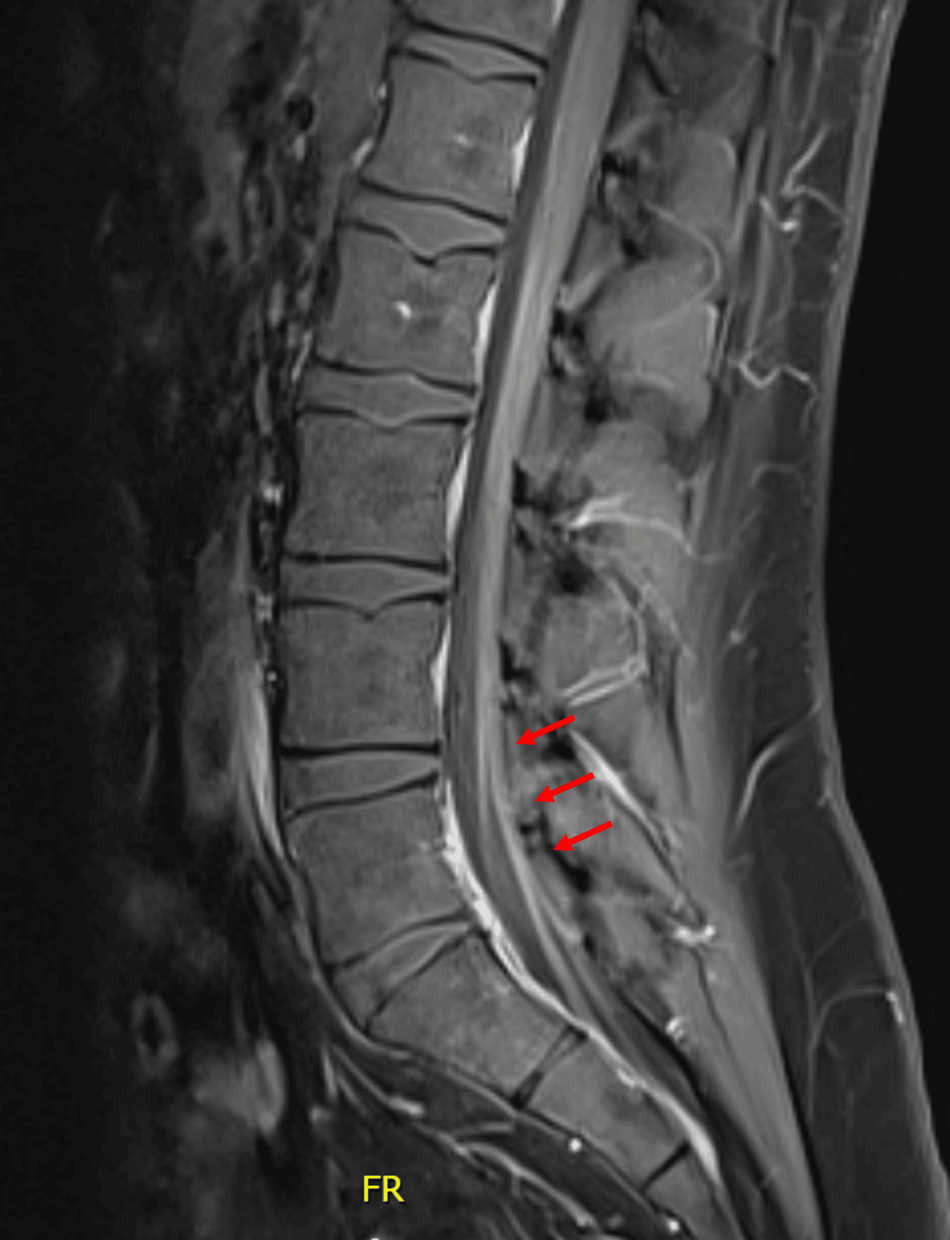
MRI with contrast of the lumbar spine (sagittal view). Note abnormal smooth enhancement along the cauda equina (arrows).
